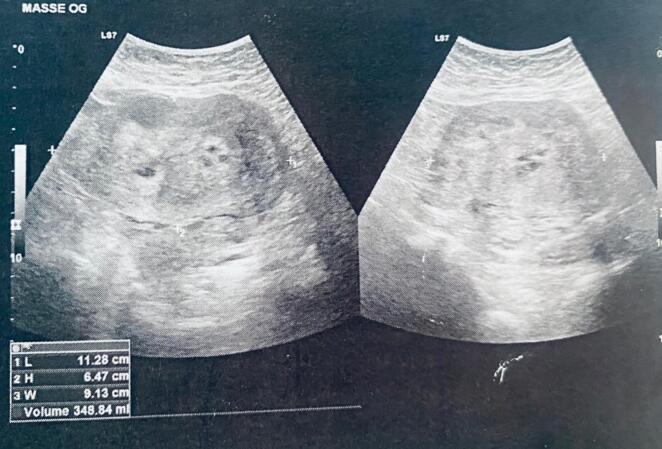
An ultrasound showing a hyperechogenic heterogeneous pre-uterine mass measuring 112 ∗ 64 ∗ 91 mm.
